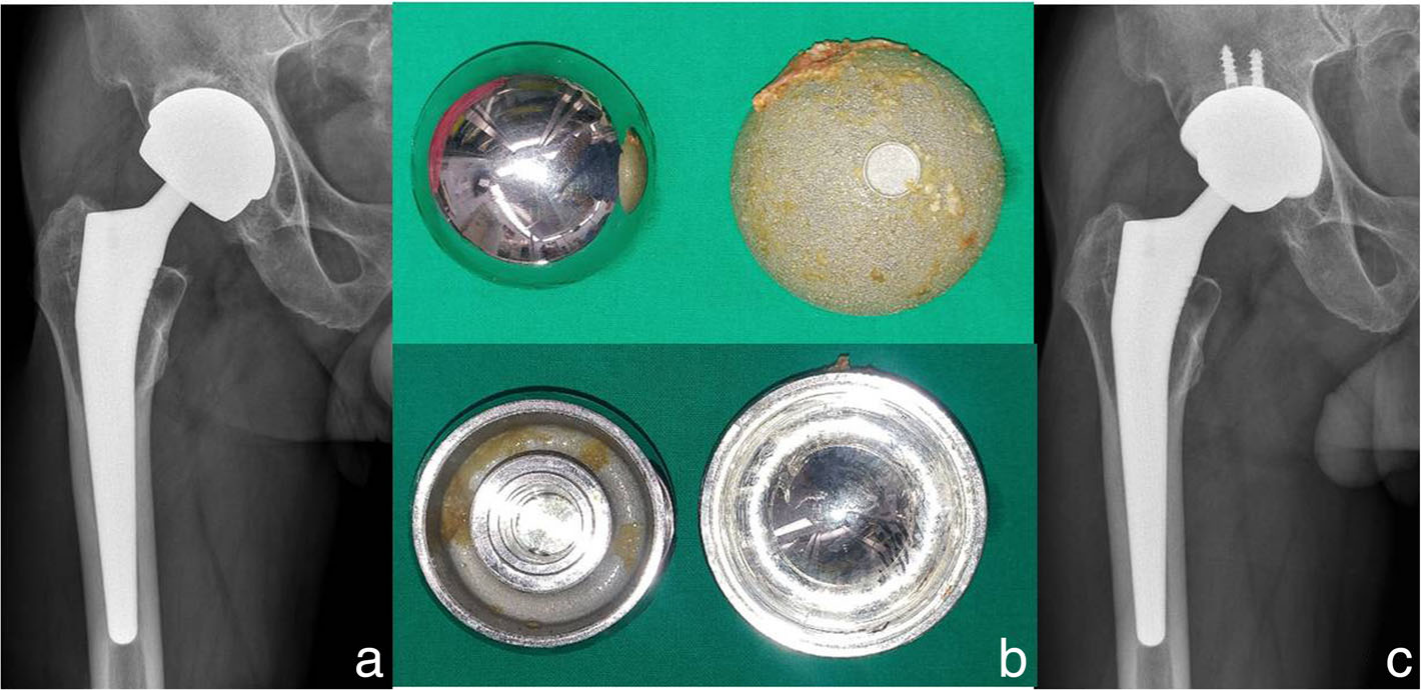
A 68-year-old male patient with right total hip arthroplasty using large head MoM bearing (a) Preoperative radiograph of acetabular aseptic loosening (b) Large head MoM bearing (c) Radiographs after acetabular revision using CoC bearing
